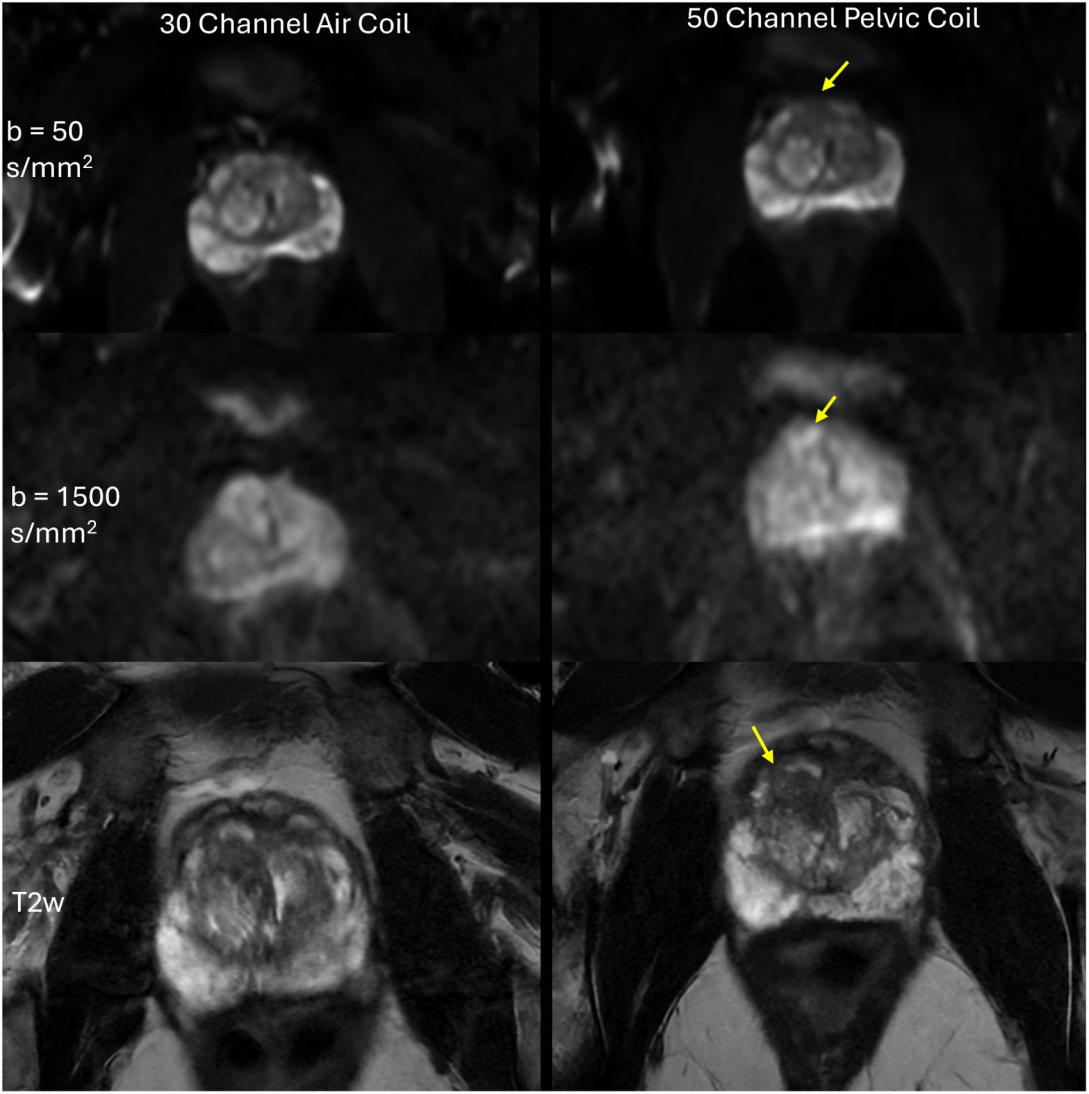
Diffusion-weighted images (DWI, top and middle) and T2-weighted (T2w, bottom) images acquired using the product 30-channel air coil and posterior array (left) and the 50-channel pelvic coil (right) are shown. All images are reconstructed with product AIR Recon DL. The patient is a 64-year-old man with a prostate-specific antigen = 5.02 ng/mL. A similar slice position is chosen. Right anterior apical transition zone Gleason 3 + 4 lesion (yellow arrow) is more well defined and hyperintense with the 50-channel coil at b = 1500s/mm^2^ (second row, right). Radiologist feedback suggests that this anterior lesion can be easily missed by urologists when using the images acquired by the 30-channel air coil.
